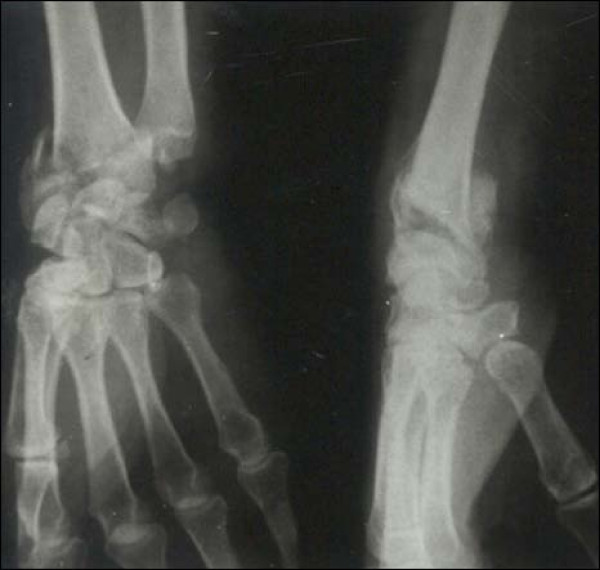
Case 2 Preoperative Radiograph Anteroposterior and Lateral view.
